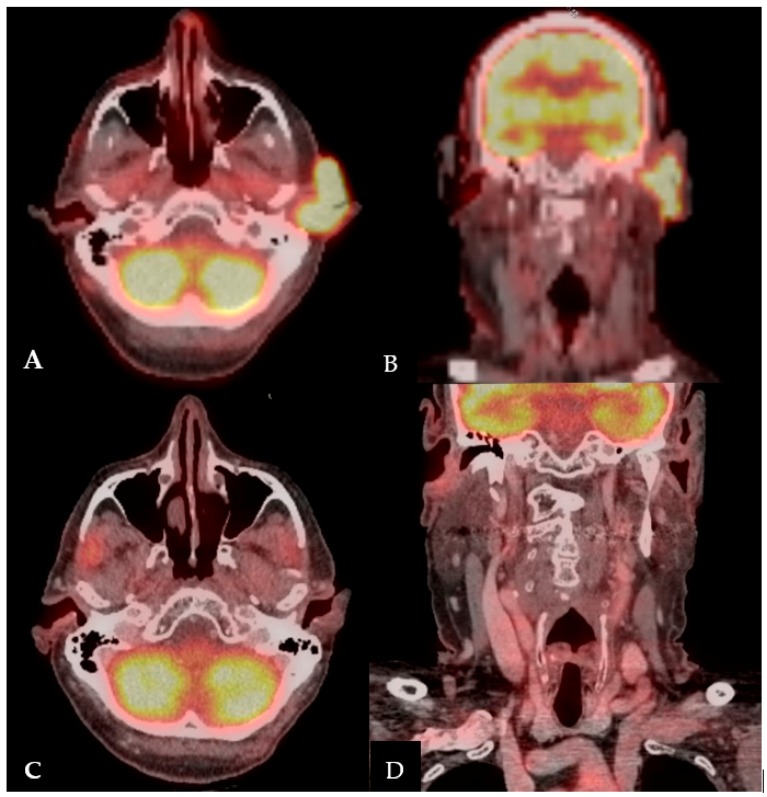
Positron Emission Tomography/Computed Tomography: (A) Axial view before immunotherapy. (B) Coronal view before immunotherapy. (C) Axial view eight months after initiating immunotherapy. (D) Coronal view eight months after initiating immunotherapy.
